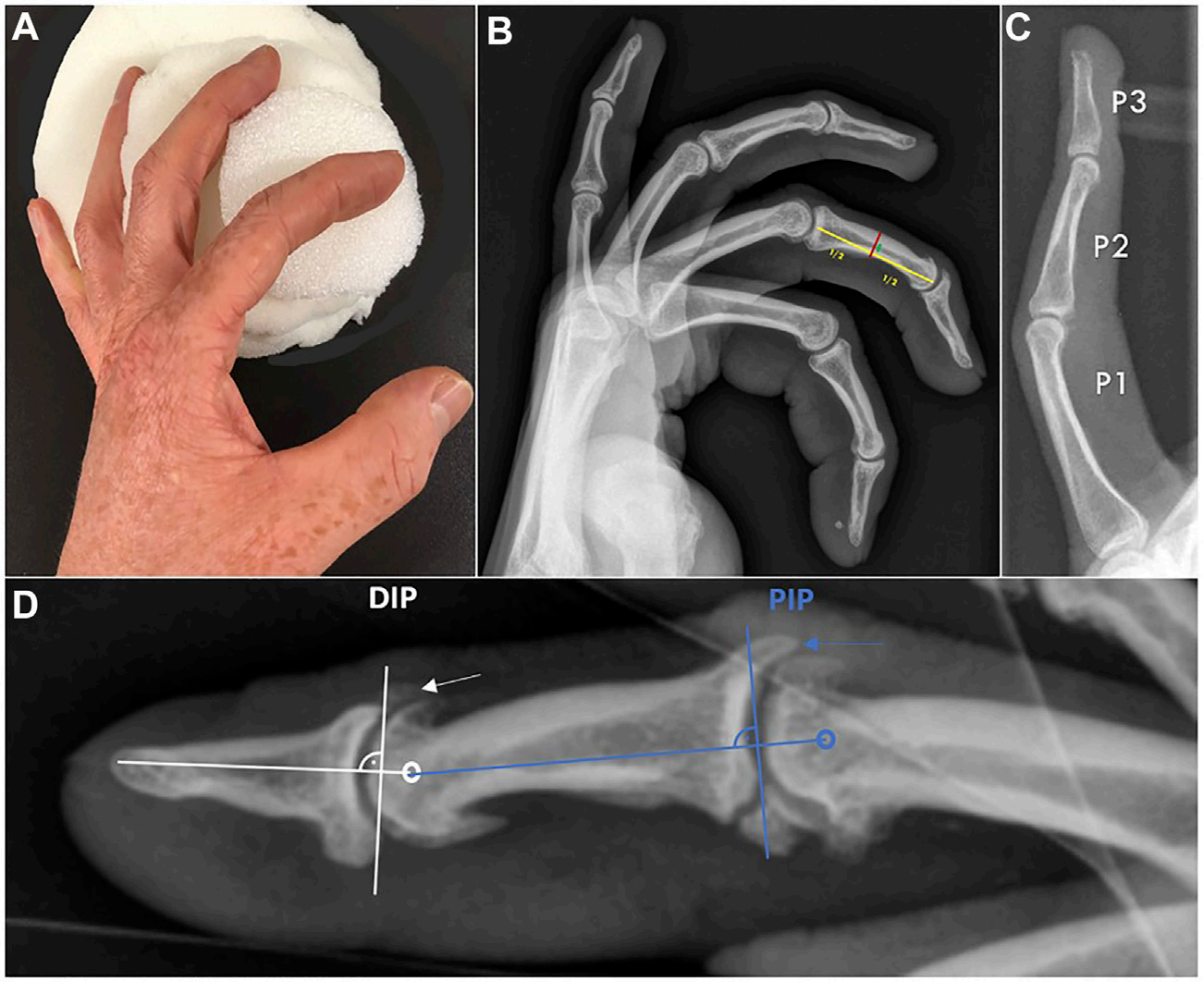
(A) To obtain standardized lateral radiographs of all fingers, a custom-made device was used. (B) Climbers: lateral radiograph of a left hand using this device. Note how all fingers are projected strictly laterally and every single cortex is visible. Measurement of the intermediate phalanx of digit III is demonstrated as an example. Red line: outer cortical width. Green line: inner cortical width. Yellow line: length of the phalanx. (C) Controls: exemplary depicted lateral radiograph of a left middle finger. Note the clear differences in cortical thickness and medullary canal width in contrast to the climber. P1: proximal phalanx, P2: intermediate phalanx, P3: distal phalanx. (D) Lateral standardized radiograph of a left digit II in a climber. Modified measurement principles according to Allenspach et al. are demonstrated. Dorsal base osteophytes were rated as present or absent at the DIP and PIP joints, respectively. The arrows mark the presence of osteophytes at the DIP and PIP joints.
